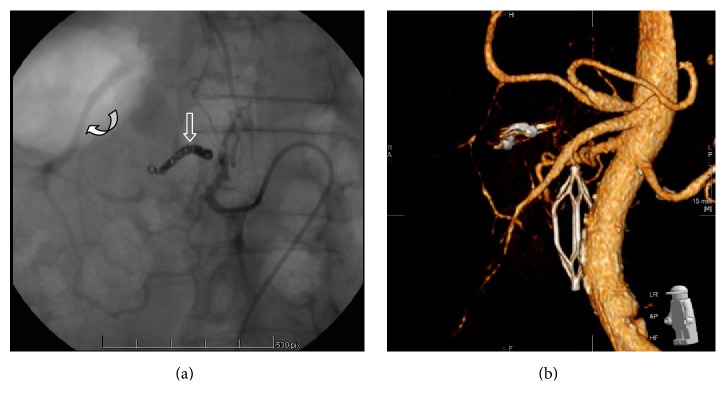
Selective angiography after coil embolization*: (a) Microcoils* (Arrow)* proximal and distal to the origin of the aneurysm. Adequate retrograde collaterals to the liver through the posterior branch are also shown* (Curved arrow)*. (b) Three-dimensional reconstruction on follow-up CT scan, showing persistent occlusion of the aneurysm, with patent hepatic artery.
